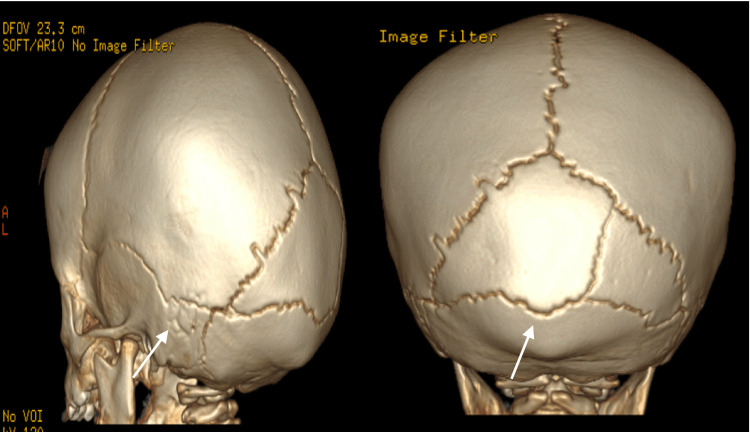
3D CT findings of a patient with accessory cranial suture in a 13-month-old male, sagittal (left) and coronal (right) views. No calvarial fracture was identified; accessory sutures separated the right lateral plate from the medial plate of the interparietal portion of the occipital bone.
